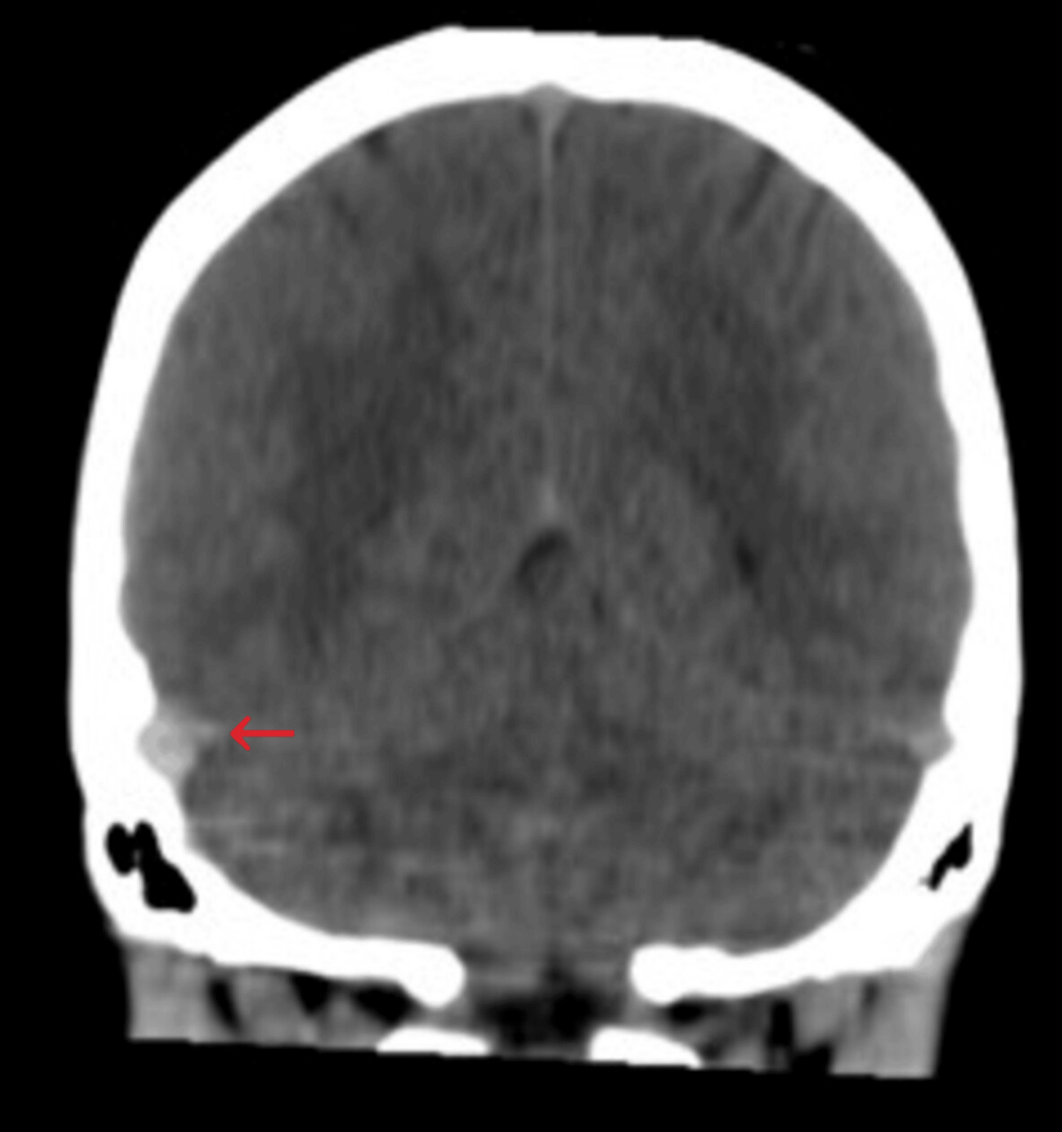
Coronal CT scan of the single skull Hyperdensity at the level of the right transverse sinus
